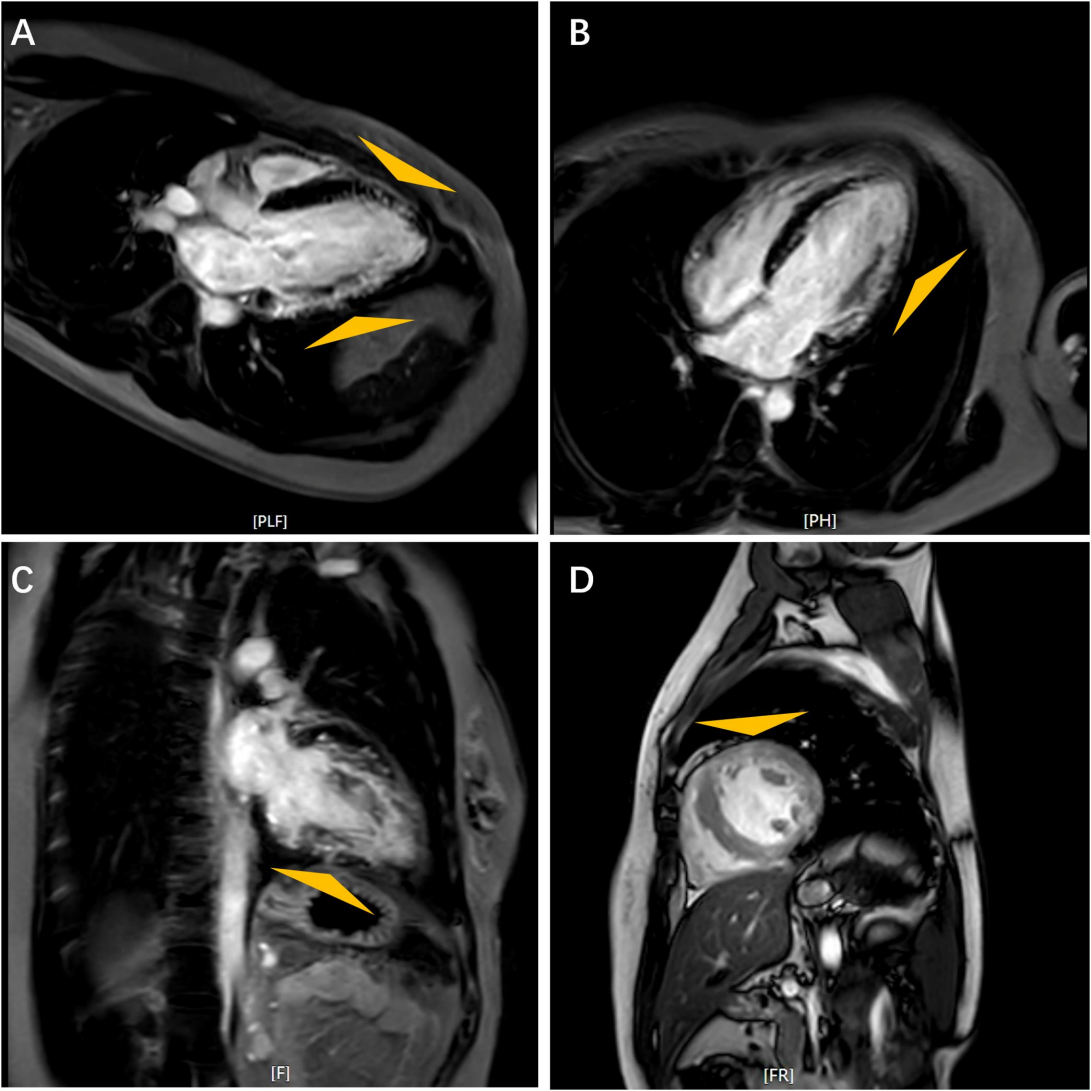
Cardiac magnetic resonance images. Feather-like delayed gadolinium enhancement in the left ventricular wall as indicated by triangles. Long Axis (A); Horizontal Long Axis (B); Four-Chamber View (C); Short Axis (D).
